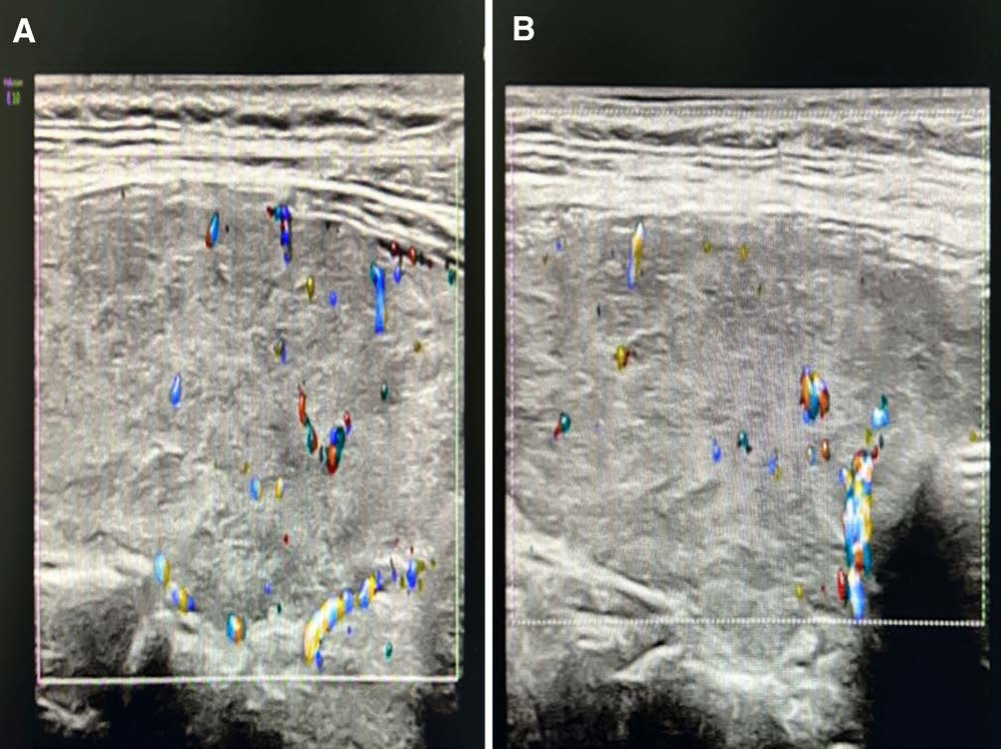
Comparison of thyroid ultrasound between the first visit and the last follow-up. (A) Thyroid ultrasound findings at the first visit. (B) Thyroid ultrasound findings at follow-up.
